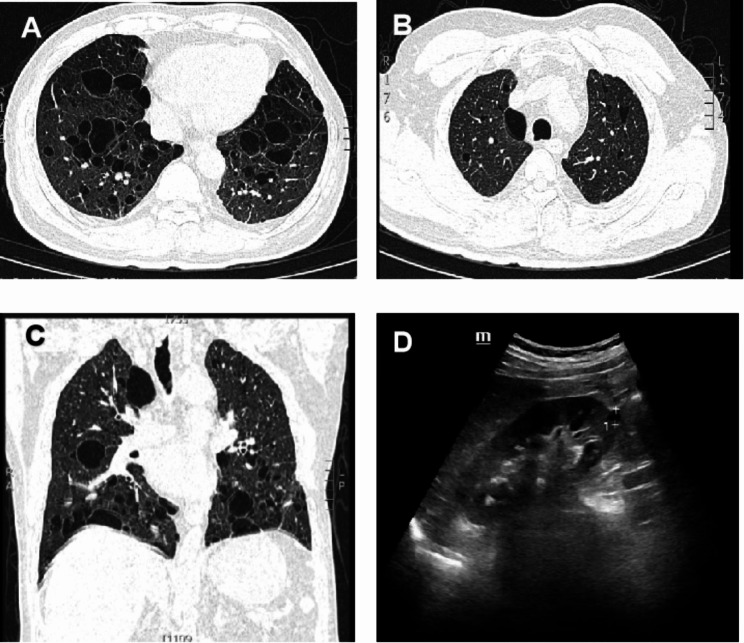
Imaging findings. (A-B) Lung HRCT shows multiple thin-walled cystic lesions throughout the lung bilateral diffuse thin walled cystic changes with a wide variation in cyst size and distribution (the biggest one > 50 mm). (C) Frontal plane reconstruction showing multiple cysts adjacent to blood vessels, fissures and visceral pleura, with predominantly basal and paramediastinal location. (D) Abdominal ultrasound indicates a right renal cyst
